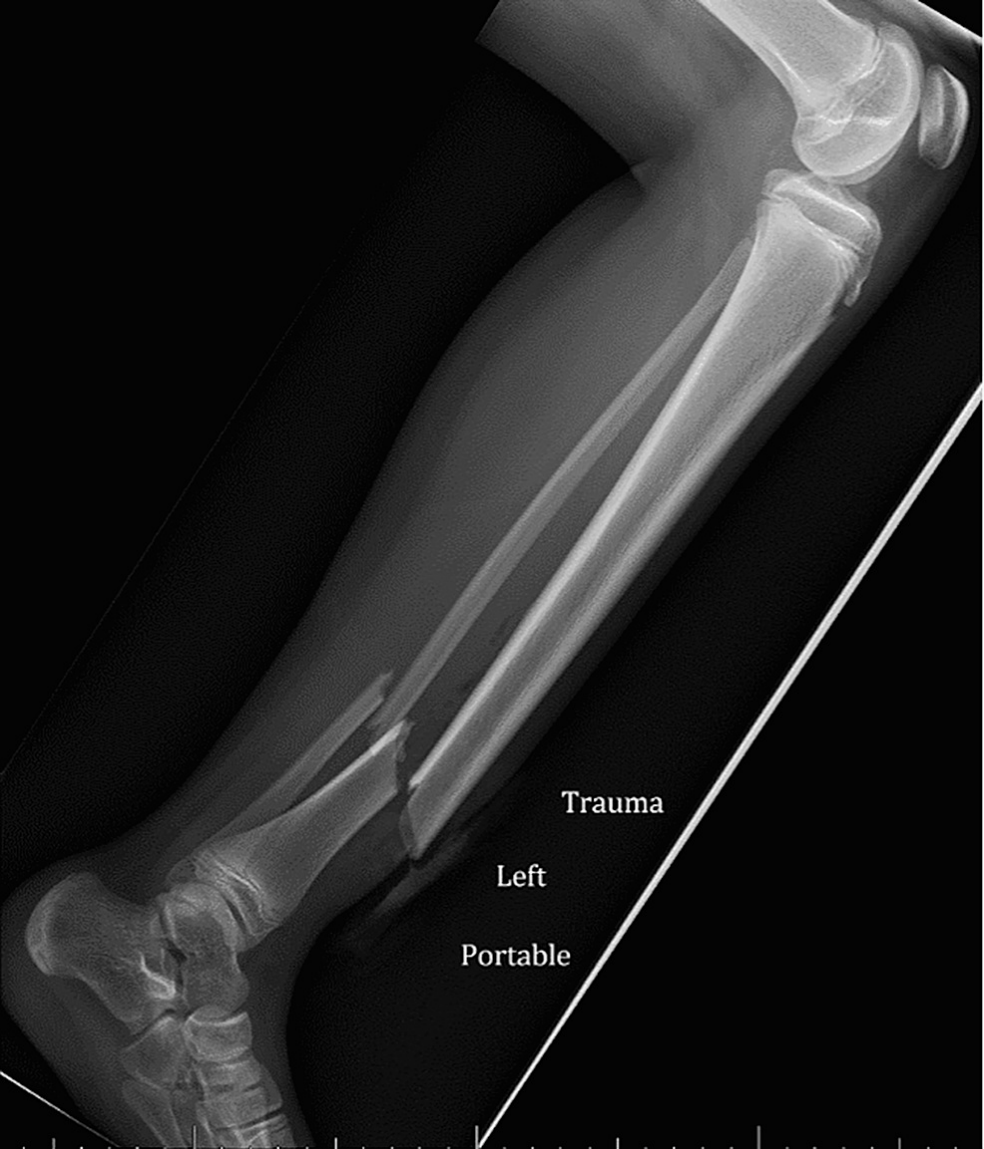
Lateral tibia-fibula radiographs of initial open tibia/fibula shaft fractures.
